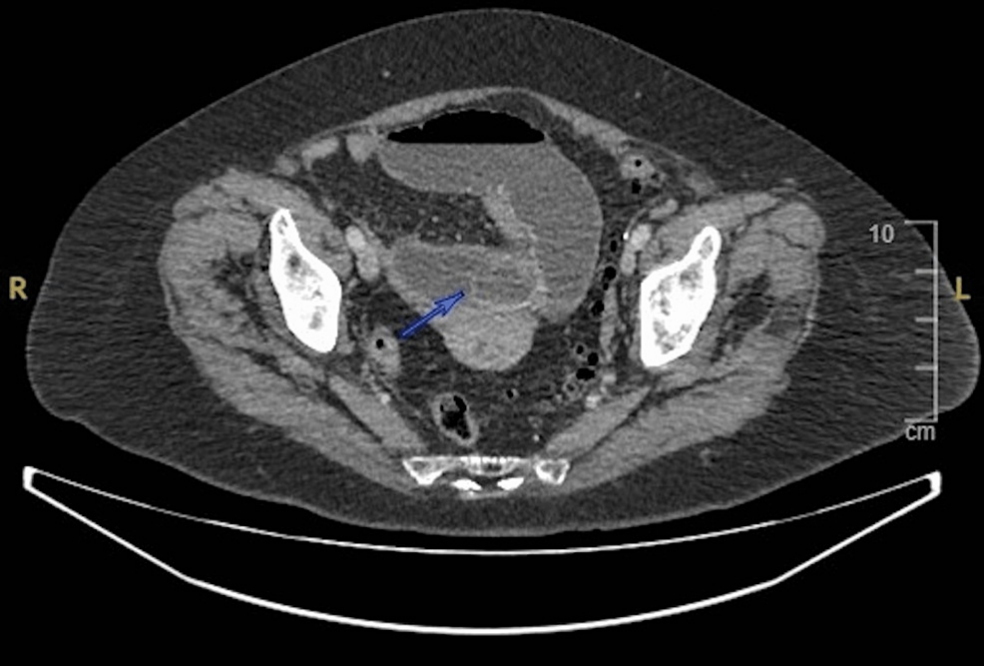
Computed tomography scan axial view of abdomen showing obstructive gallstone in pelvic loop of mid ileum with features of small bowel obstruction.
